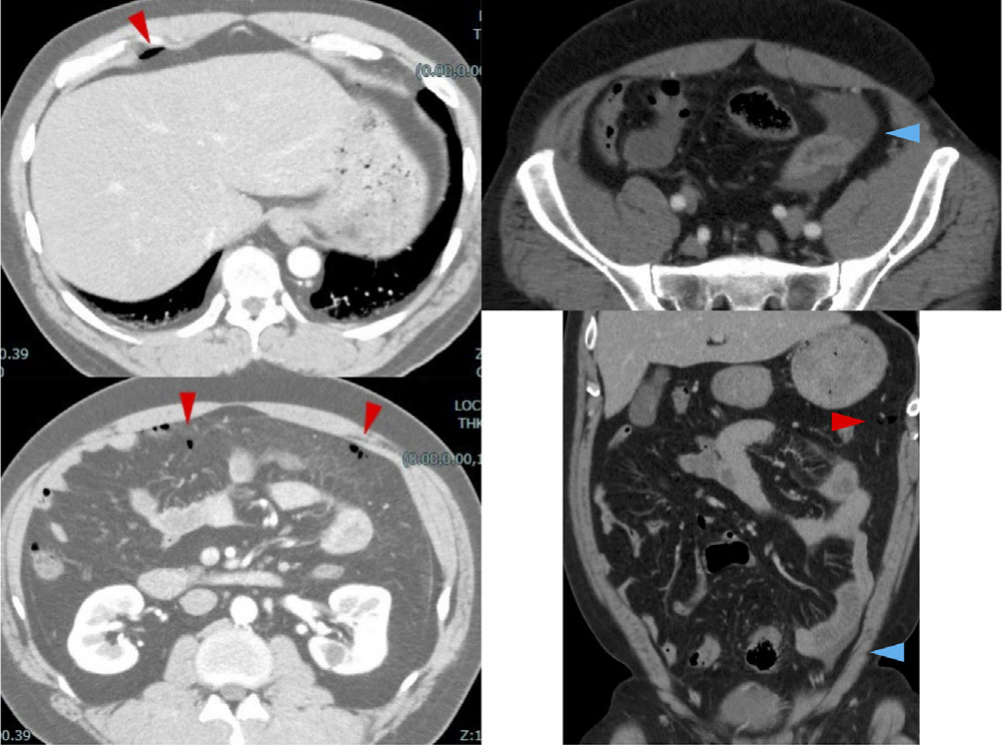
Contrast‐enhanced abdominal computed tomography at our hospital. Red arrows indicate free air. Blue arrows indicate ascites.
